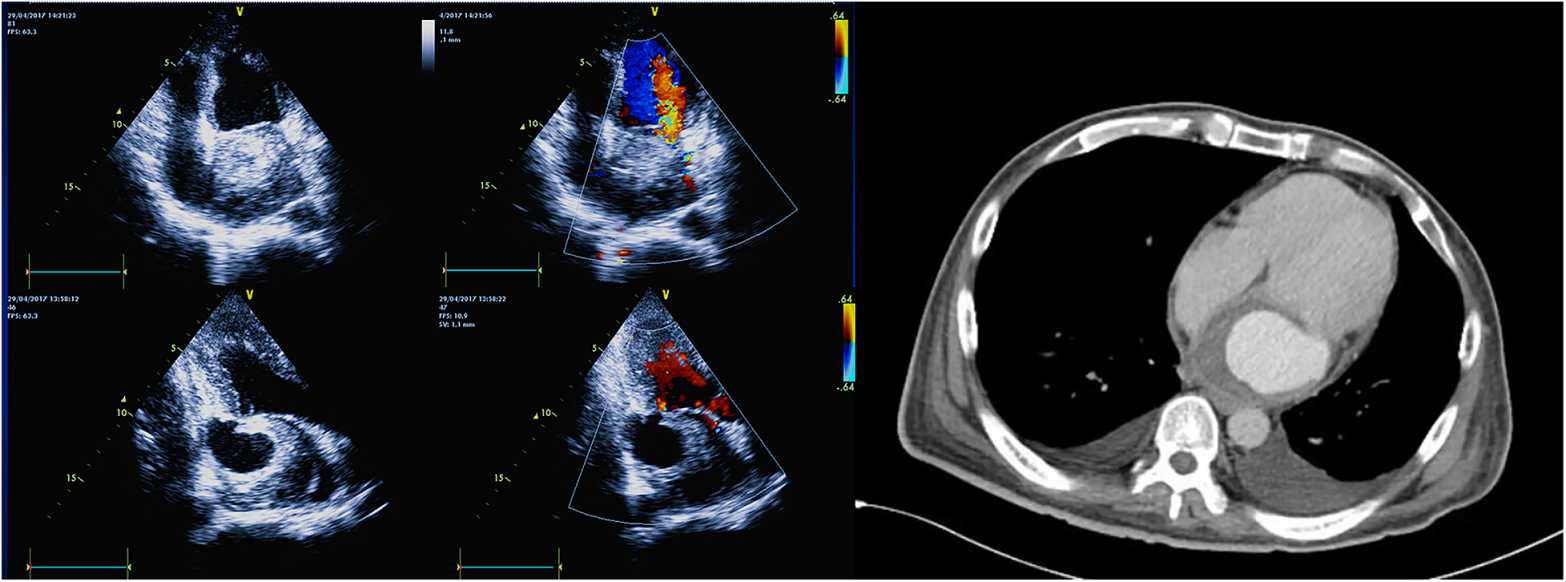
TTE showed a mass containing thrombus in the LA and a suspicious endocardial orifice beneath the root of the PML on the LV. CT revealed contrast filling the mass cavity.
